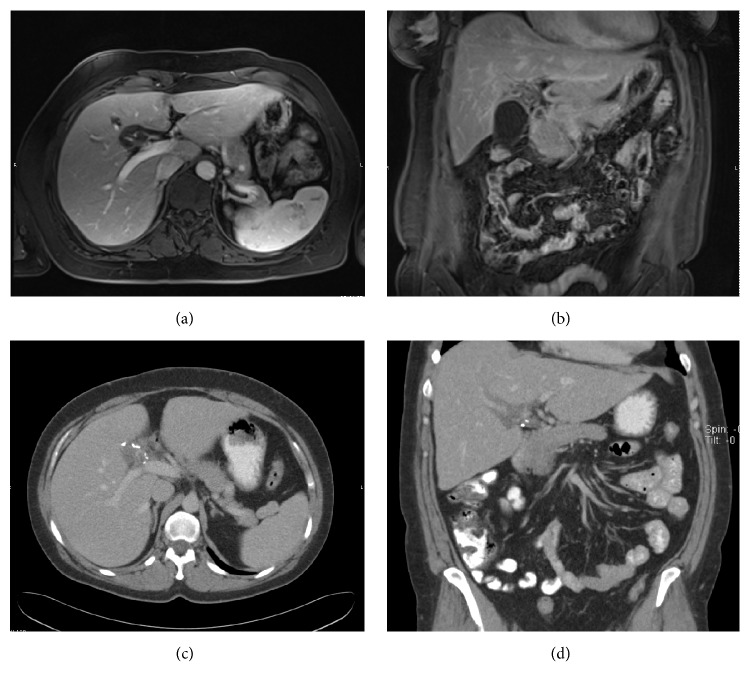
(a and b) Representative axial and coronal images from contrast enhanced preoperative MRI of the patient. Encasement of hepatic artery with extension of the mass predominantly towards the right side is noted in GB fossa. (c & d) Follow-up postoperative CT scans 5 years later depicting almost stable appearance of the mass.
